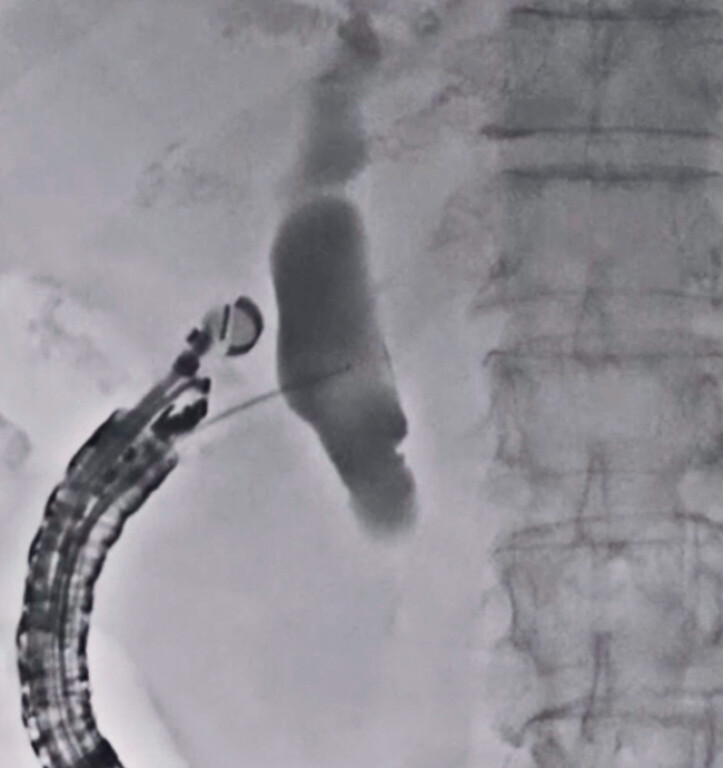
At endoscopic ultrasound-guided choledochoduodenostomy (EUS-CDS), the common bile duct is punctured using a 19-G needle and the contrast medium is injected.
